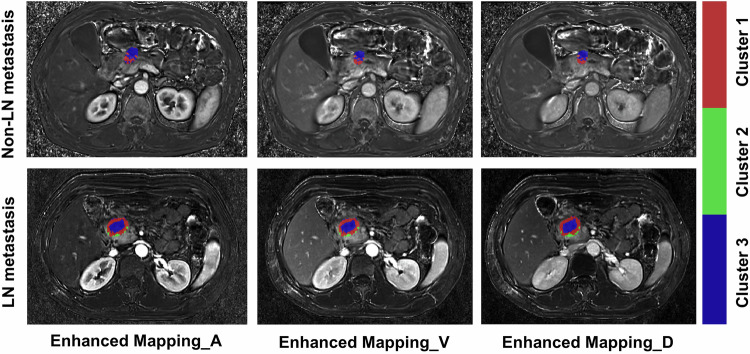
Representative MRI images of patients with LN-positive and LN-negative metastasis in Enhanced Ratio Mapping_arterial (Enhanced_A), Enhanced Ratio Mapping_venous (Enhanced_V), and Enhanced Ratio Mapping_delayed (Enhanced_D) sequences. Each color displays a distinct tumor habitat
